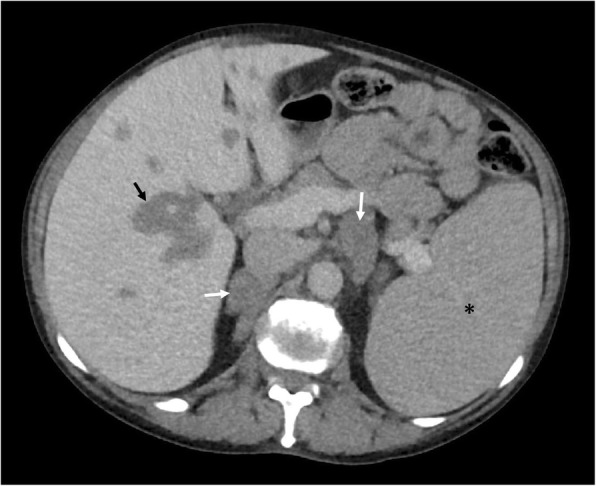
Axial CT of the abdomen demonstrates symmetrical bulky and homogenous low attenuating masses in the adrenal glands (white arrows), consistent with extramedullary haematopoiesis in the adrenal glands. Note also similar attenuation bulky perihilar soft tissue consistent with periportal extramedullary haematopoiesis and splenomegaly (asterisk)
